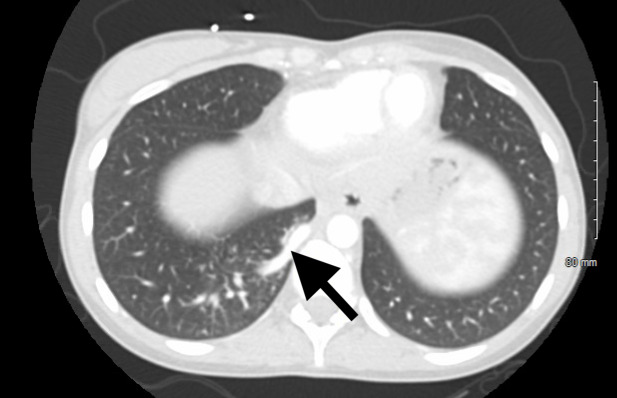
There is no evidence of pulmonary embolism or aortic dissection on the computed tomography angiogram. Arrow shows anomalous pulmonary artery originating from descending aorta.
